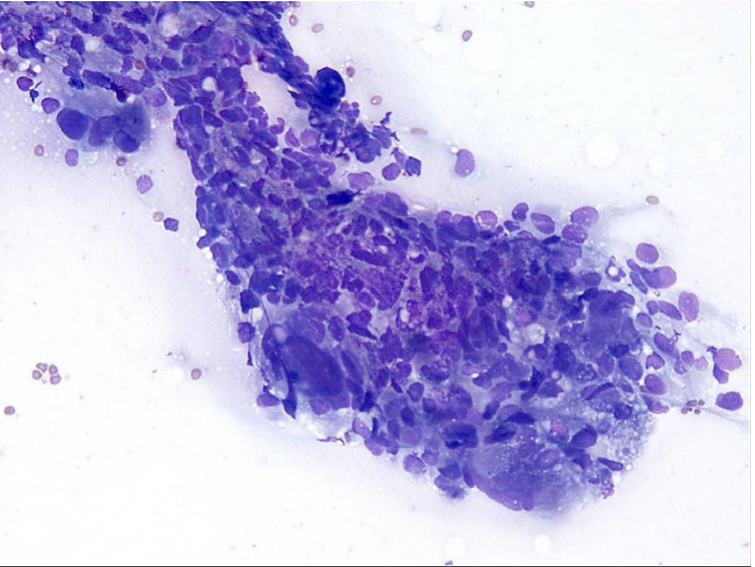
CT-guided FNA of anterior peritoneal mass, showing a somewhat cohesive large cluster of highly pleomorphic cells (Diff-Quik, ×200).
